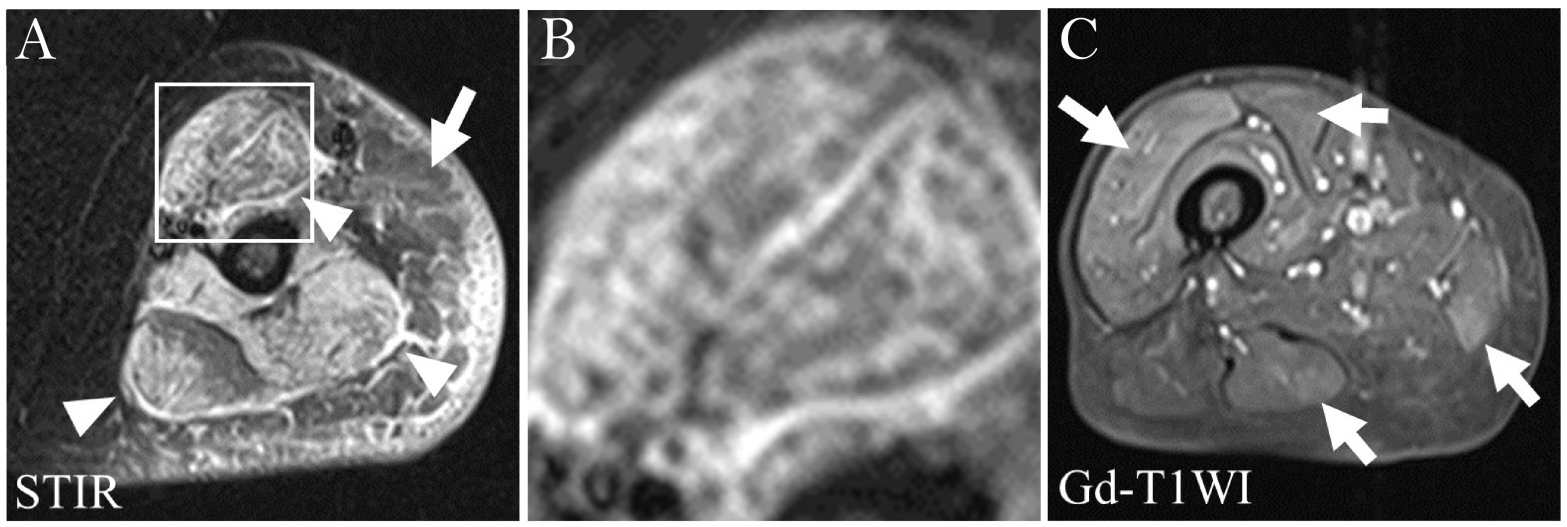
(A) STIR image of the left upper arm of a patient with DM. Structures with HSI are the subcutaneous adipose tissue (arrow), fasciae (arrowheads) and muscles. Distribution and pattern of HSI in the biceps muscle are diffuse and honeycomb, respectively (within the square). (B) Higher magnification image of the square in (A). (C) Gd-T1WI image of the right thigh of a patient with PM. There is diffuse HSI in the vastus lateralis muscle and patchy HSI in the rectus femoris, gracilis and semitendinosus muscles, producing a foggy pattern (arrows). DM, dermatomyositis; Gd-T1WI, gadolinium-enhanced fat suppressed T1-weighted imaging; HSI, high signal intensity; PM, polymyositis; SHSI, strong high signal intensity. Figure adapted with permission from Ukichi et al. (31).
